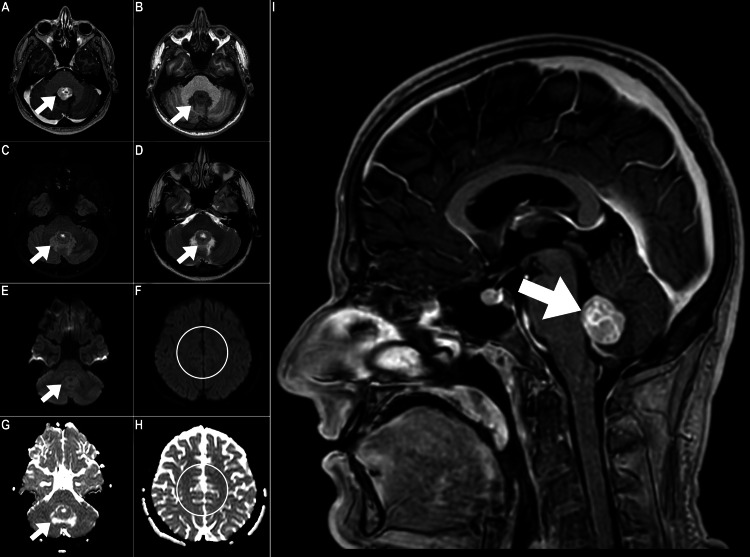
Follow-up brain MRI. (A) Follow-up axial T1-weighted image post gadolinium contrast medium administration, (B) axial T1-weighted pre-gadolinium contrast medium administration, (C) fluid attenuation inversion recovery (FLAIR) axial sequence, (D) T2-weighted axial brain MRI sequence, (E, F) diffusion-weighted images, (G, H) apparent diffusion coefficient maps, and (I) sagittal T1-weighted image post-gadolinium contrast medium administration was performed three weeks later. The mass within the fourth ventricle shows an intermediate signal on T1- and T2-weighted sequences with avid contrast enhancement and a central small cystic component. The mass shows marked enlargement over short intervals (measuring about 3 cm vs 1.5 cm previously) with moderate edema surrounding the fourth ventricle appreciated on T2-weighted and FLAIR sequences.  No proximal ventricle dilatation was seen. The diffusion-weighted images and apparent diffusion coefficient map at the lesion and centrum semiovale showed no diffusion restriction within the mass.
